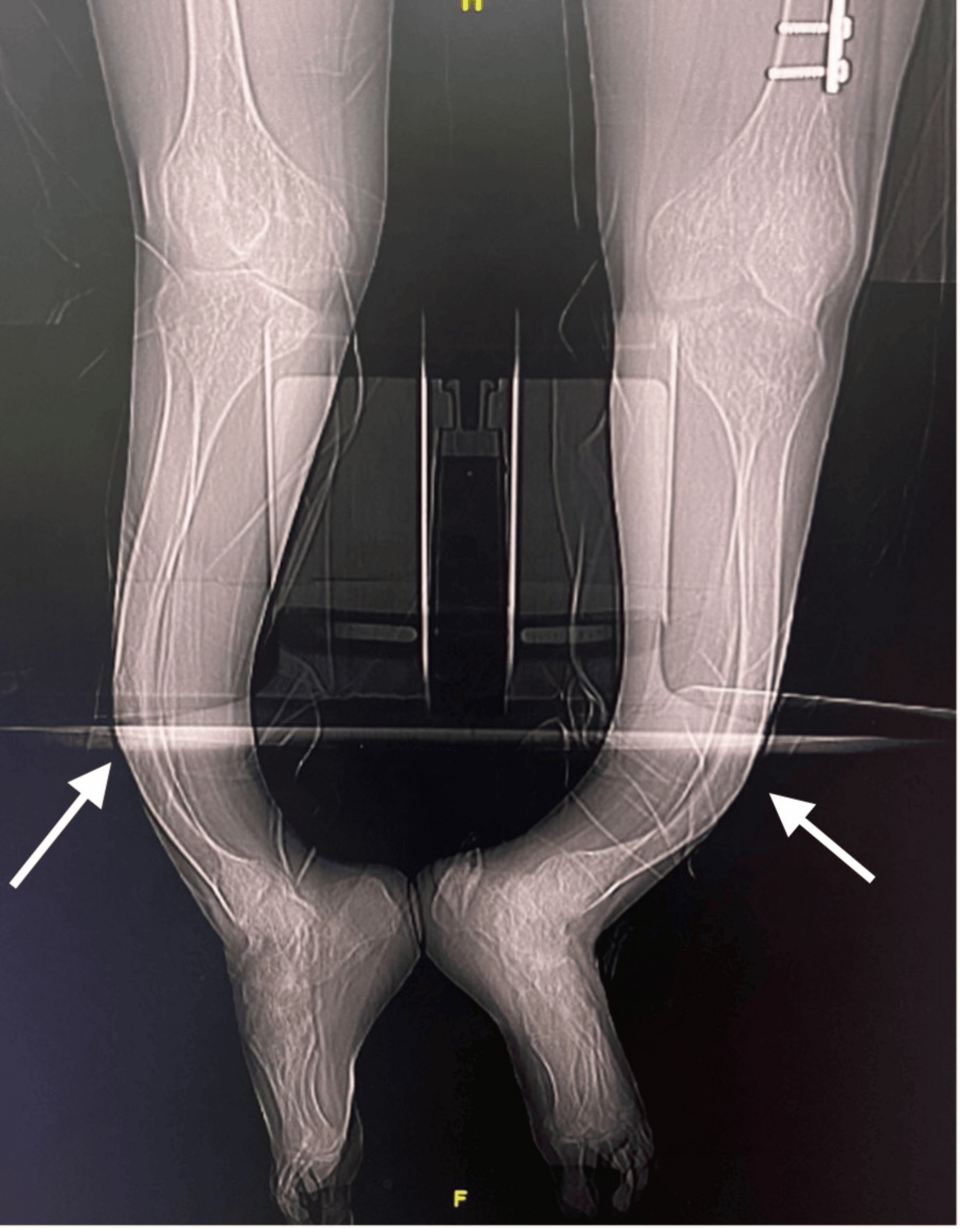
Preoperative CT scan showing severe bilateral anterior bowing of the bilateral tibia (arrow)
